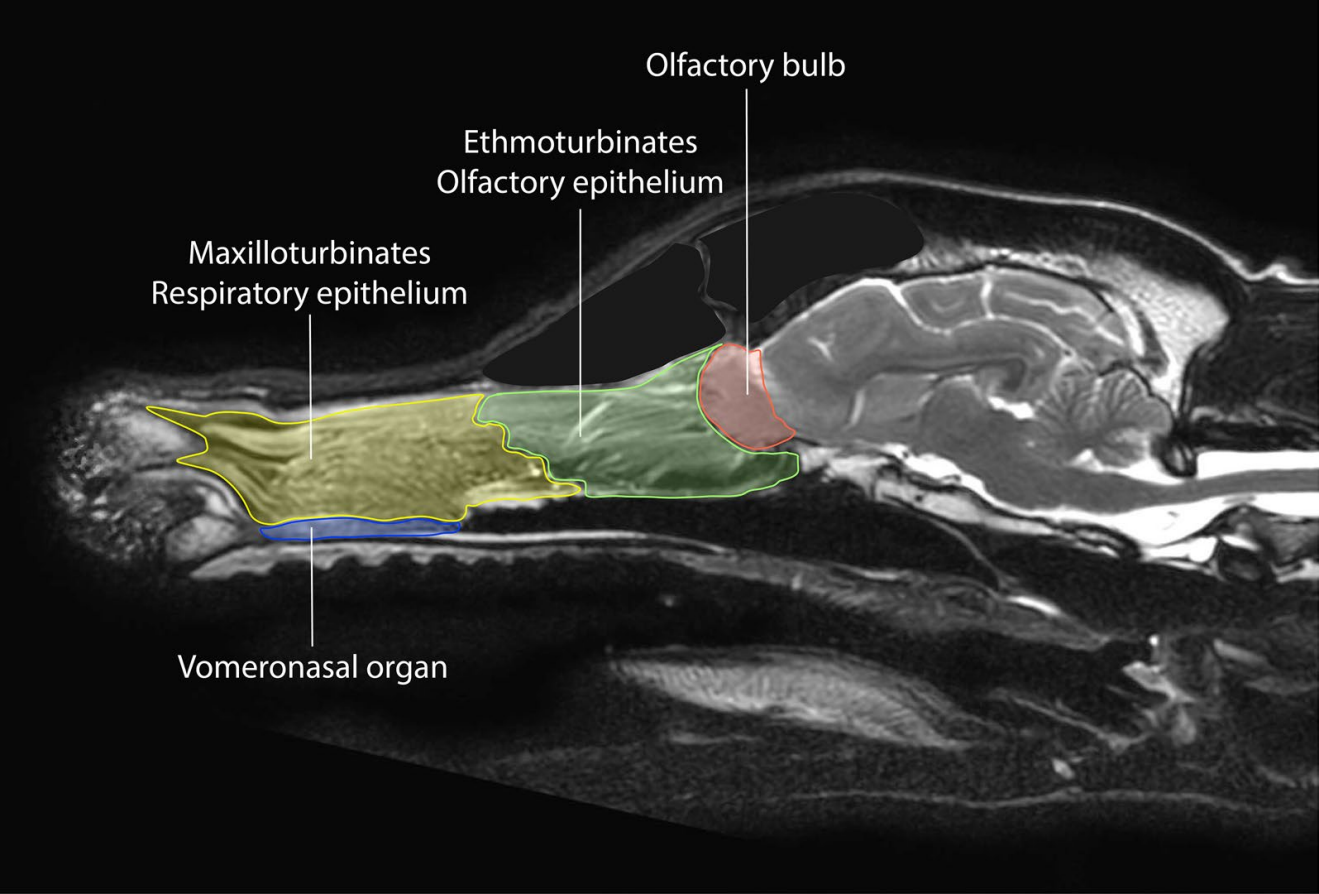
Sagittal magnetic resonance imaging highlighting the inner structures of the olfactory system. The blue area represents the vomeronasal organ, the respiratory epithelium in the maxilloturbinates is highlighted in yellow, the olfactory epithelium in the ethmoturbinates near the lamina cribrosa is shown in green, and the red area contains the bulbus olfactorius
